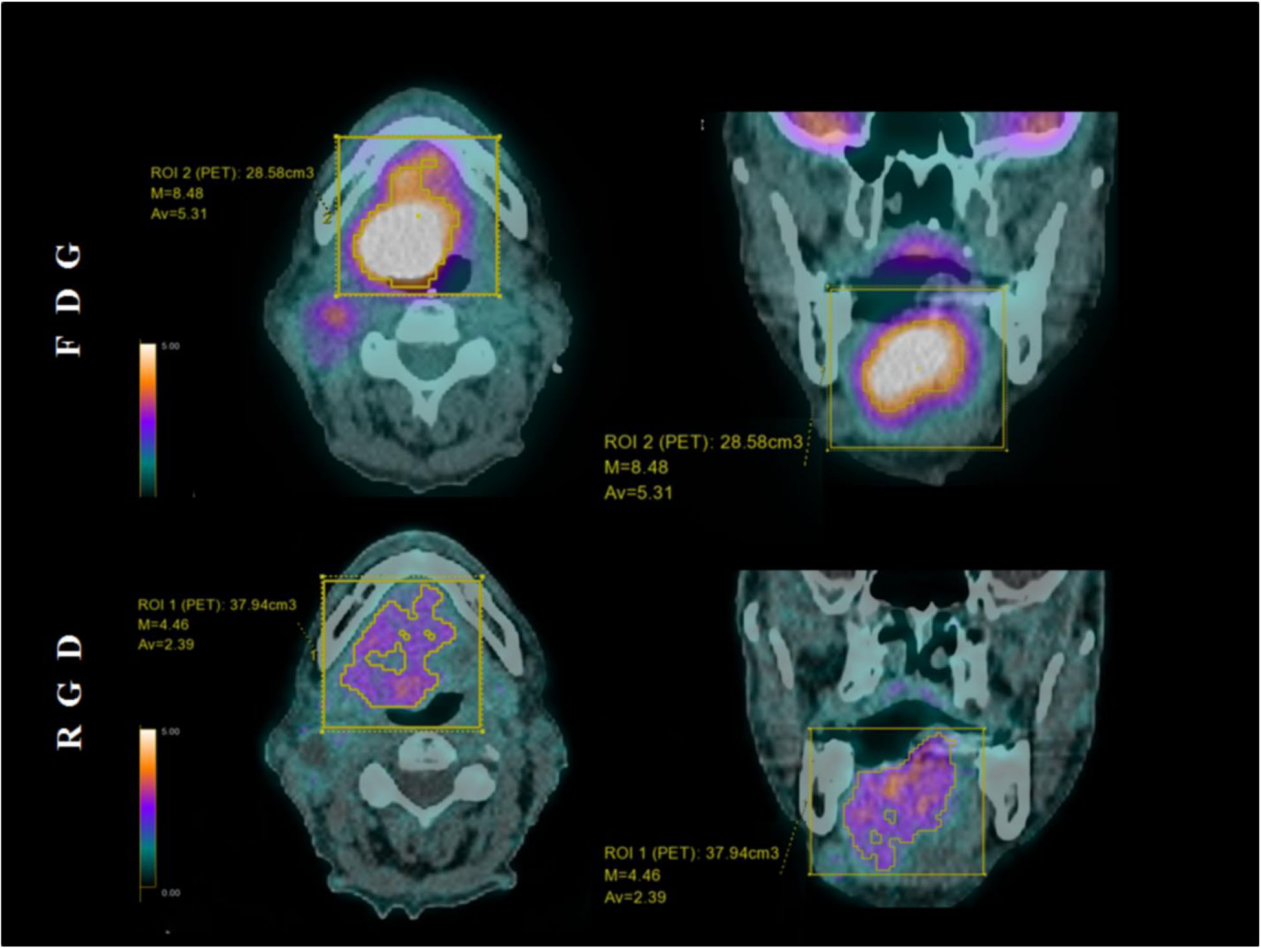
Example of axial and coronal ^18^F-FDG and ^68^Ga-NODAGA-RGD PET/CT 3-D volume of interest semi-automatically delineated on a 42%  SUV_max_ threshold for patient #8 using a parallelepipedal bounding box
